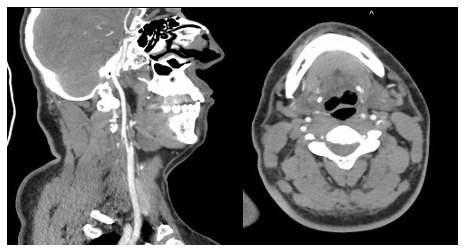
contrast-enhanced computed tomography (CT) scan showed in the cervicothoracic section a thrombosed dissection of left internal carotid.
